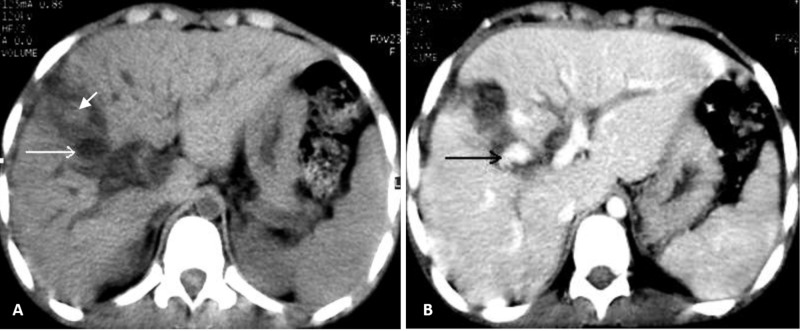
Hepatic axial CT scan. This hepatic axial CT scan without (A) and with (B) contrast showed a hypodense defect in the anterior segment of the right lobe with a slight spontaneously hyperdense blood clot (A, short arrow). The presence of a well-defined hypodense (A, long arrow) and enhanced formation (B, long arrow) suggested a pseudoaneurysm. Note the slightly dilated intrahepatic bile ducts.
